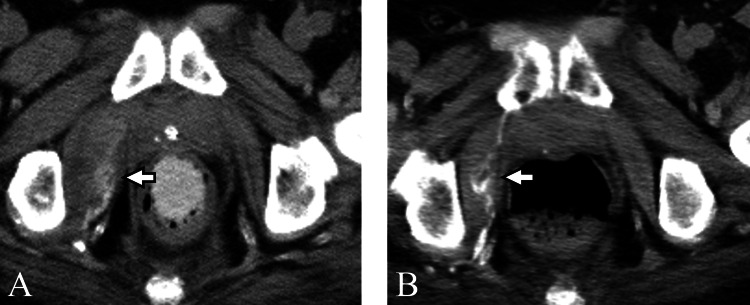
Case two A 63-year-old man with myositis ossificans in the right short external rotator muscles. (A) On the 28th day of hospitalization, CT performed for evaluation of fever demonstrated swelling accompanied by calcification in the right muscles (arrow). Manual muscle testing score in the right lower limb was 0. Pneumonia was identified as the cause of the fever. (B) On the 97th day, follow-up CT showed the development of ossification with a decrease in the swelling (arrow).
